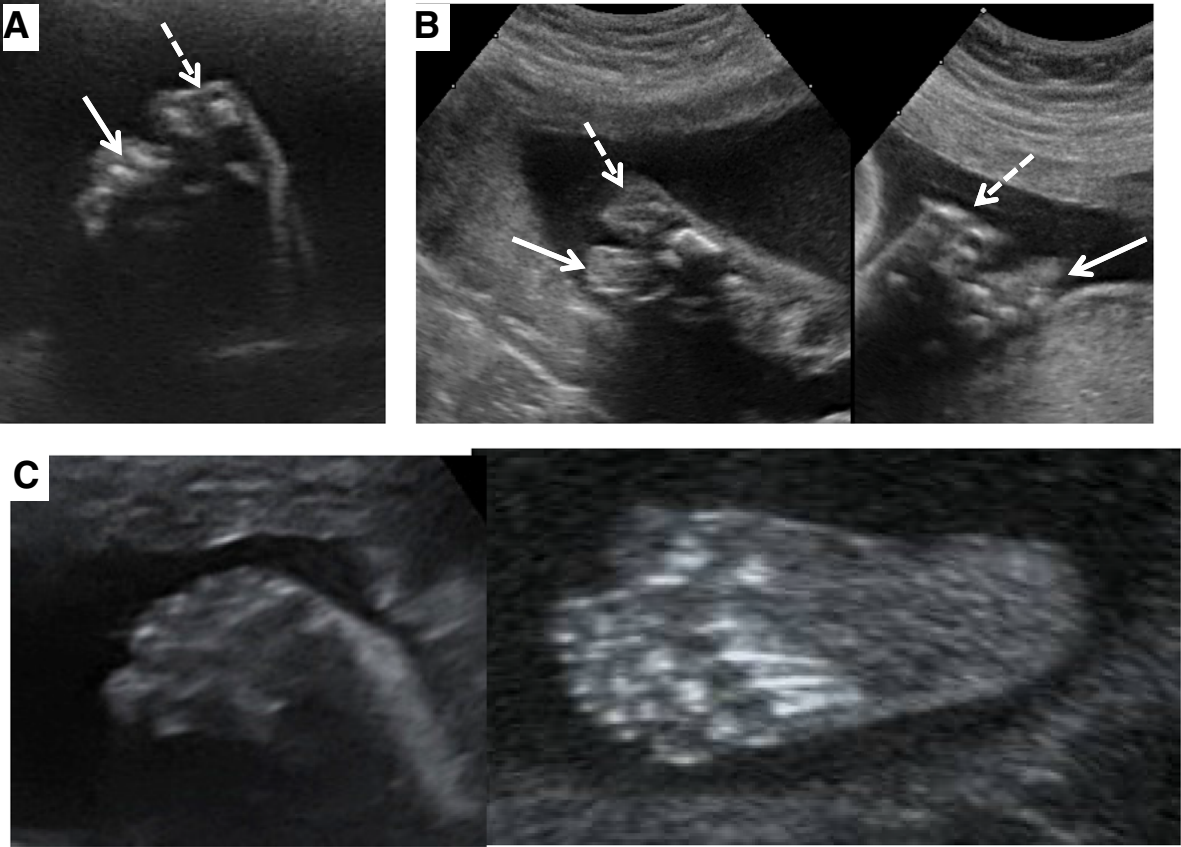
Ultrasound examination of the feet of the fetus with the p.R254W *FGFR1* mutation at 23 weeks of gestation. a and b: Ultrasound views respectively of the soles of the right and left feet of the fetus carrying the p.R254W FGFR1 mutation. The dashed white arrow shows the syndactyly of toes I and II, and the solid white arrow that of toes III and IV. c Plantar ultrasound view of a normal fetal foot
